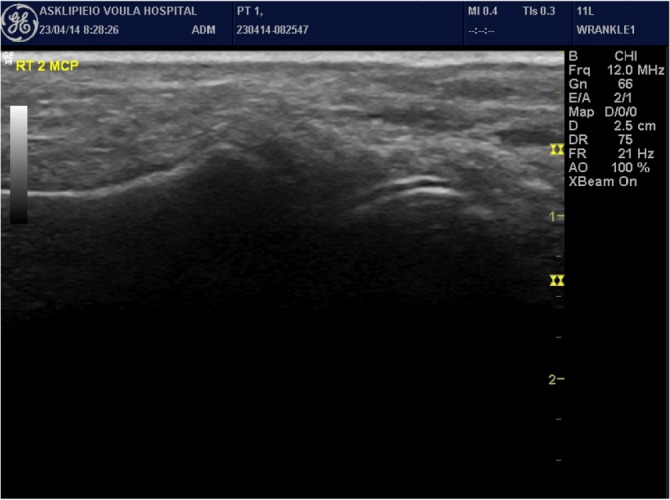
Patient 2. Ultrasound of metacarpophalangeal bone with double contour sign.
